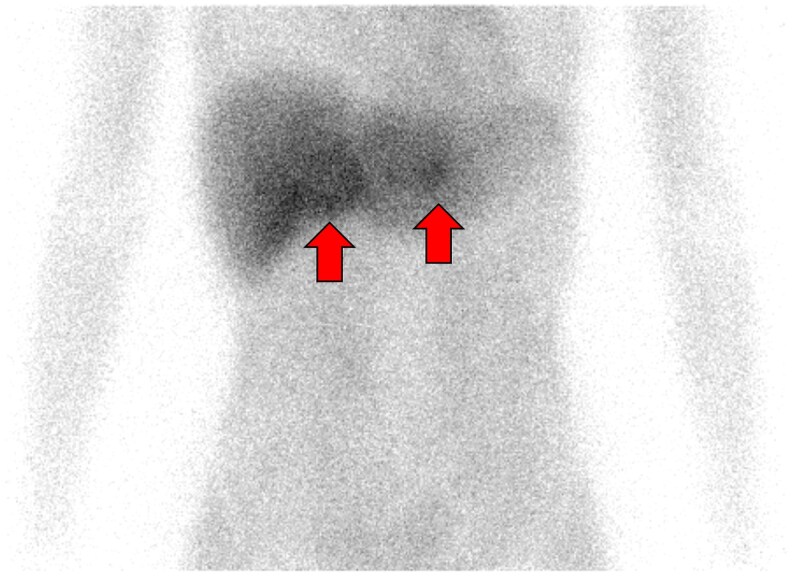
^123^I-meta-iodobenzylguanidine (MIBG) scintigraphy. Enhanced accumulation of MIBG in the adrenal masses was observed bilaterally.
